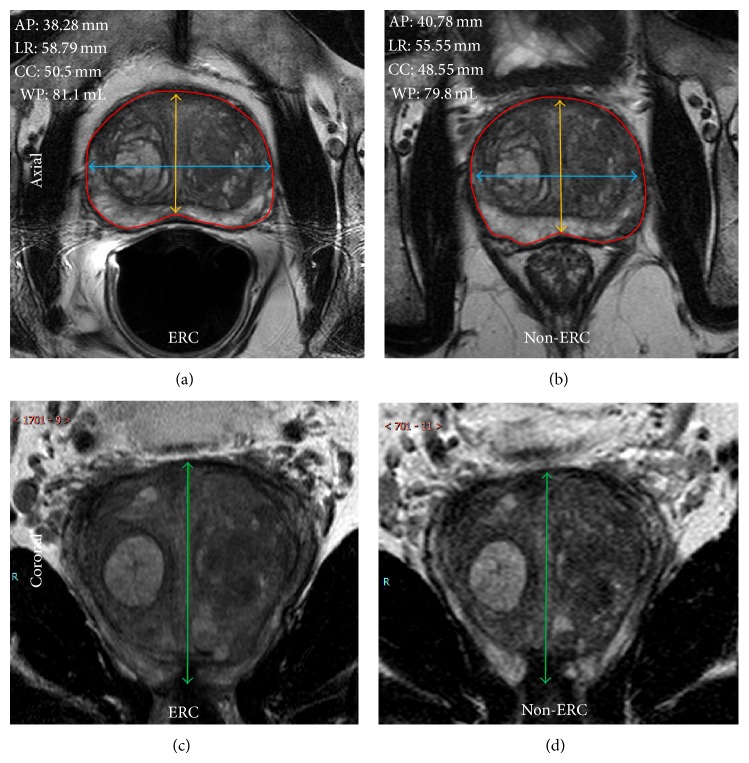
Axial ERC and non-ERC T2W MRI depicting the maximum AP (yellow) and LR (blue) prostate dimensions and WP contours (red) used for volume determination are shown below. Coronal ERC and non-ERC T2W MRI of the same patient were used to measure maximum CC (green) prostate dimension. AP, LR, and CC measurements are also annotated.
